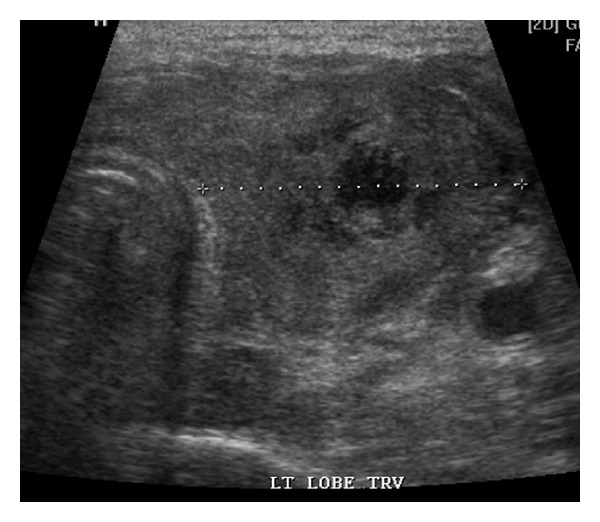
Thyroid sonogram showing a 3.7 × 2.5 × 2.9 cm oval heterogeneous structure, in the left lobe.
